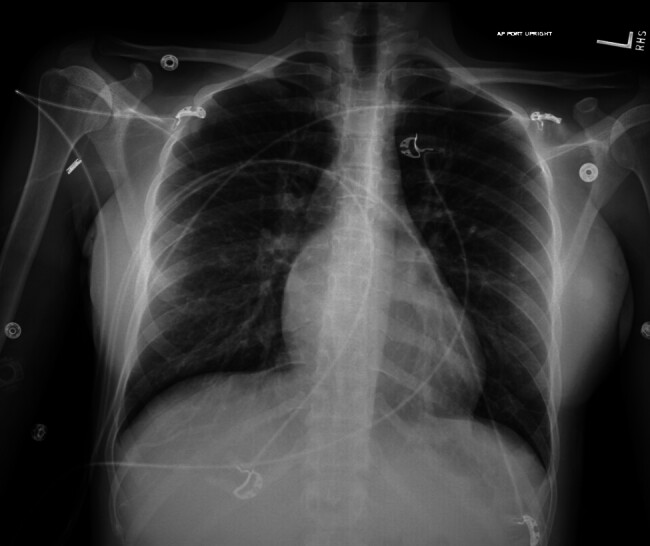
Negative chest X-ray at the time of partial hydatidiform mole diagnosis.
